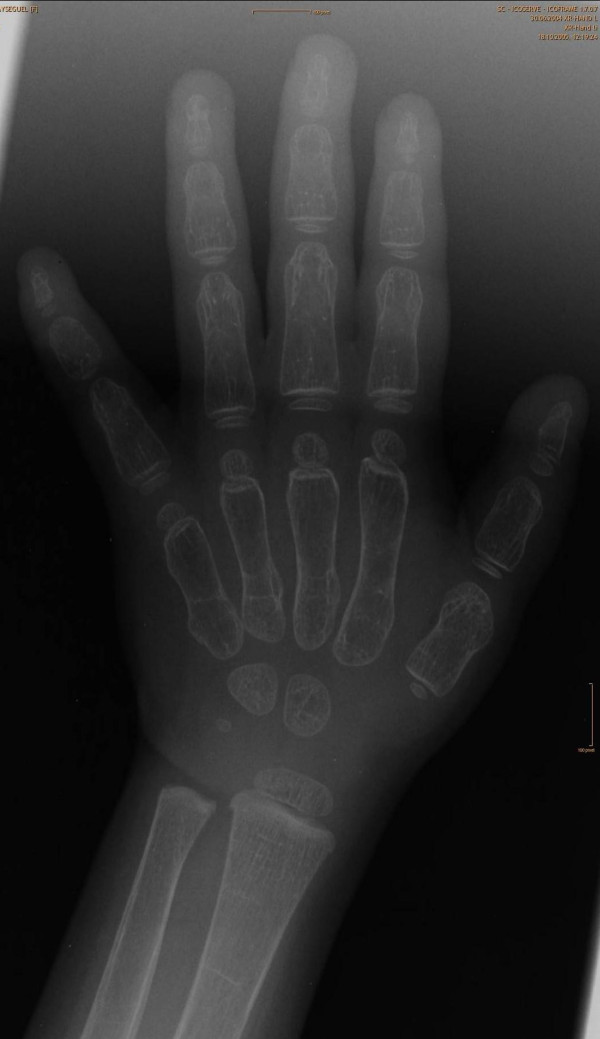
Anteroposterior hand radiograph at 10 years showed 2.2 years bone age. Marked widening of the distal portions of the metacarpal metaphyses and diaphyses respectively associated with thinning of the cortices and osteopenia.
